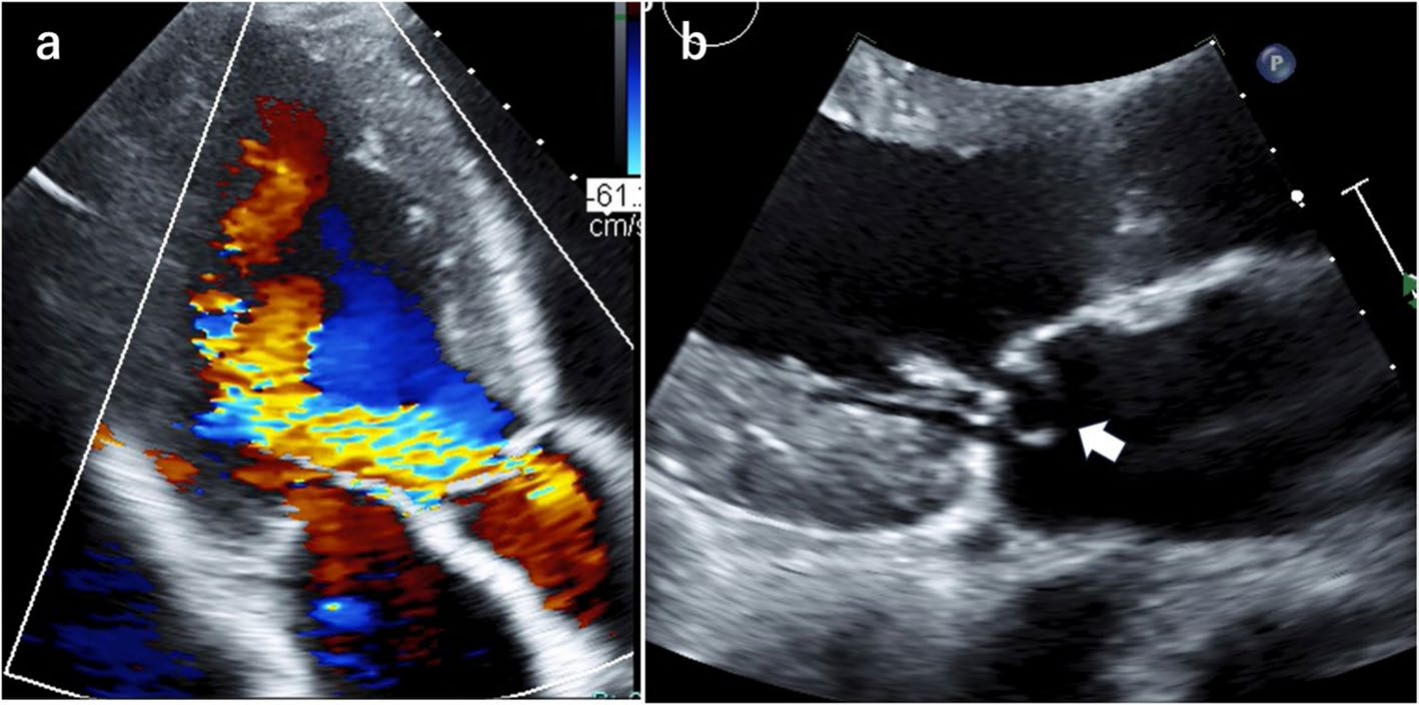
Transthoracic echocardiograms showing severe eccentric aortic regurgitation (a) and an anterior mitral valve leaflet aneurysm (white arrow) (b)
